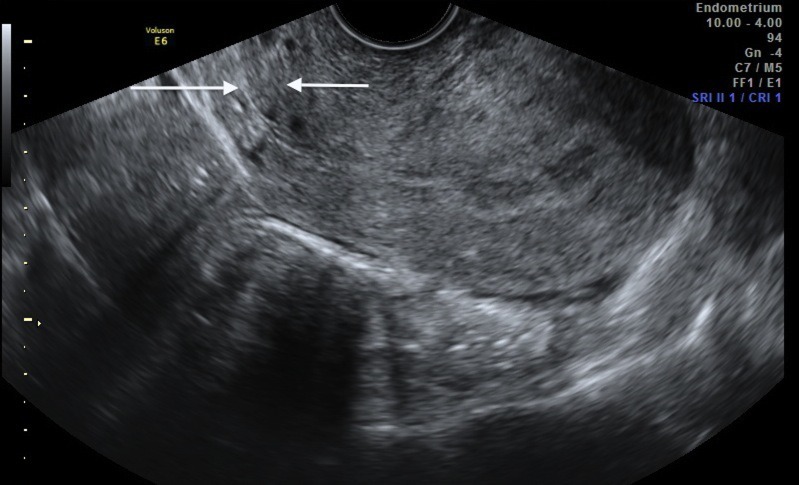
Transvaginal ultrasound displaying one internal cervical os (black arrow)
